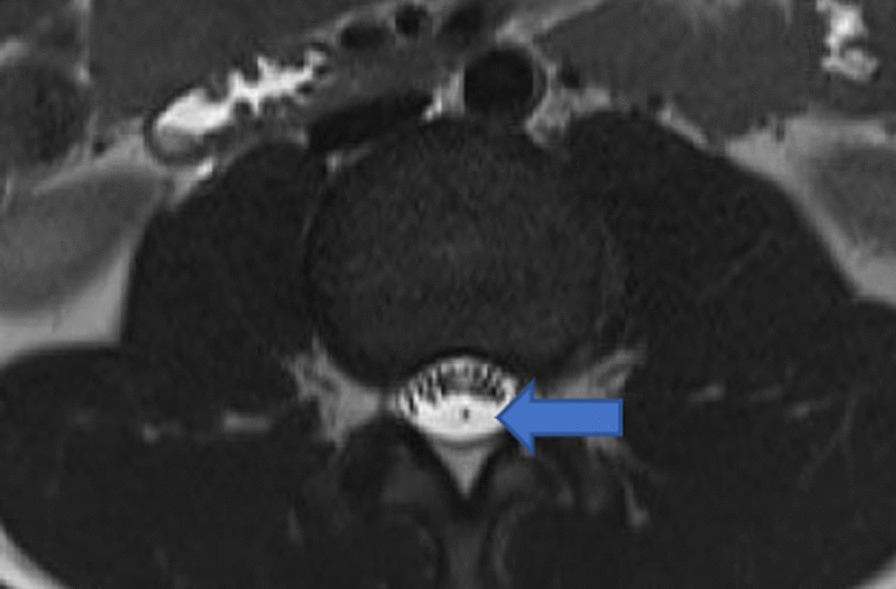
MRI (T2-weighted) of the lumbar spine in the supine position. The filum terminale and cauda equina are separated (arrow)
